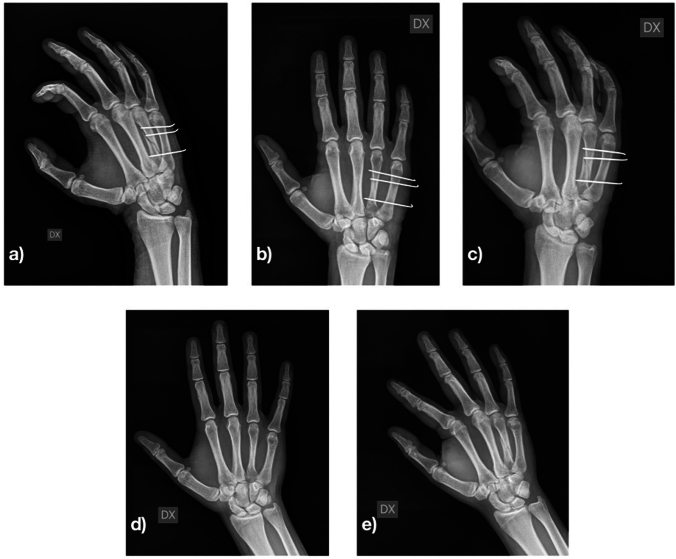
Fourth metacarpal spiral fracture. a post-operative radiography; b and c 1-month post-operative radiography; d and e 3-month post-operative radiography
